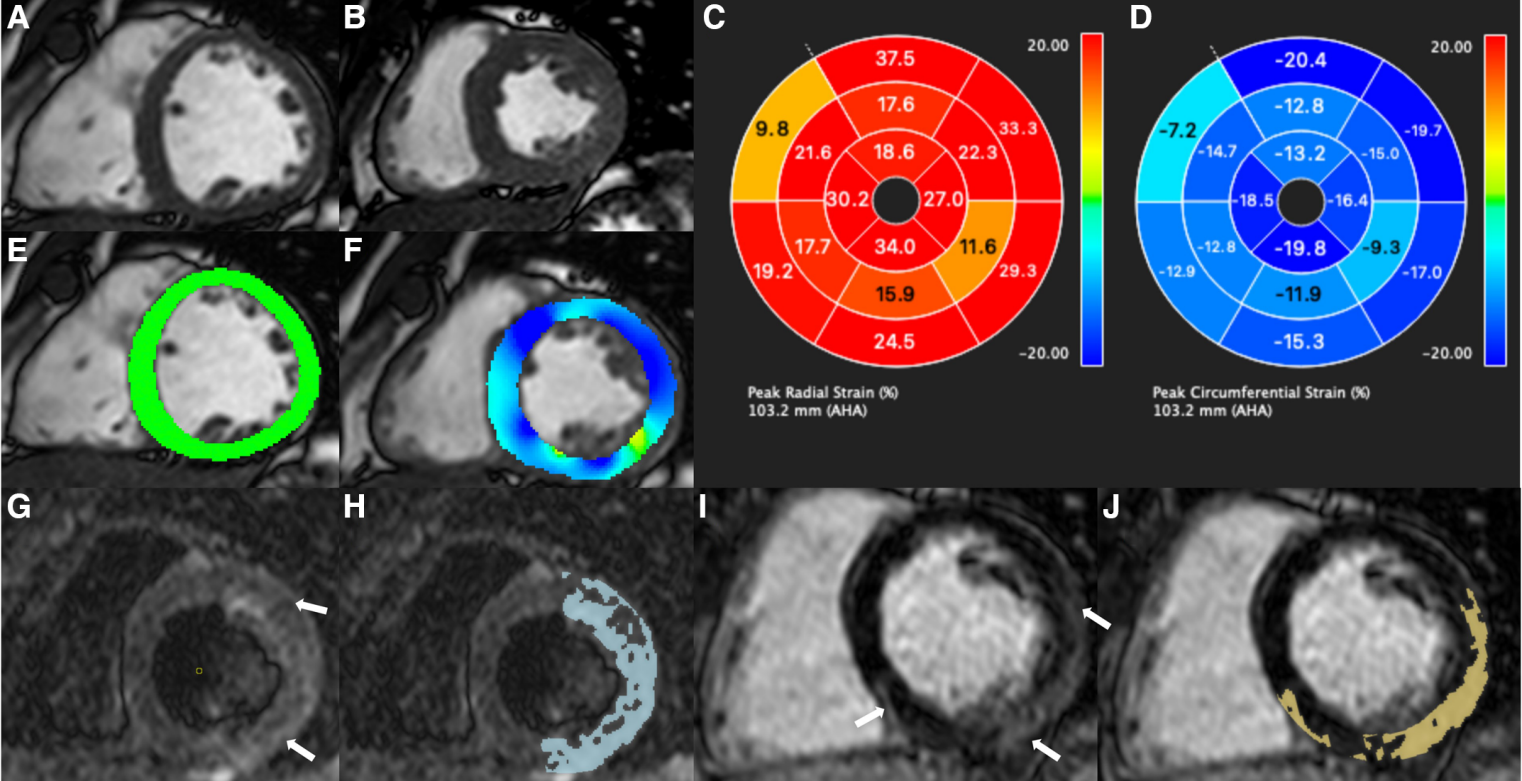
In (A,B) cine short axis in medial cut in diastole (A) and systole (B). (C,D) Bullseye radial strain and circumferential strain, respectively. The endocardial and epicardial were delimited manually in diastole in short-axis cine images in medial location of the left ventricle with automatic propagation to the entire cardiac cycle. Circumferential strain in the short axis cine in medial location of the LV in diastole (E) and systole (F) with contractile defect in the inferior, inferolateral and attenuated contraction in the septal and anterior region, corroborating with the bullseye (C,D). In (G) T2-weighted image in medial section of the LV showing edema (white arrows) and quantification (blue areas). Image (I) showed delayed short-axis enhancement with myocardial fibrosis (white arrows) and quantification (yellow areas).
